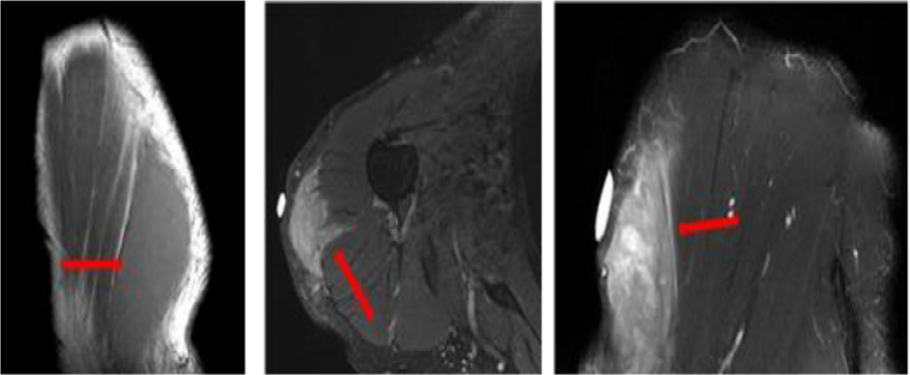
(A) Sagittal T1, (B) axial PDFS, and (C) Coronal T2FS MRI of the right shoulder demonstrate a solid heterogenous soft tissue mass involving the lateral belly of the deltoid muscle and extending laterally into the subcutaneous fat, over the area of palpable abnormality as demarcated by the external marker (red arrows). The lesion was mildly hyperintense to skeletal muscle on T1-weighted images, and more pronouncedly hyperintense to skeletal muscle on T2-weighted images.
